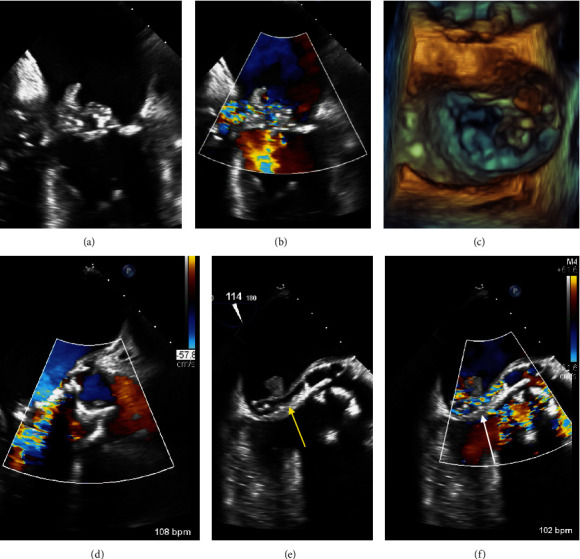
Transesophageal echocardiogram obtained approximately two weeks into hospital stay. (a–c) Bulky vegetation on the mitral valve extending along the aorto-mitral curtain with associated mitral regurgitation and possible leaflet perforation that are not well-characterized. (d) High-velocity flow in the LVOT concerning for regurgitation, as well as (e) an abnormal lucency along the aorto-mitral curtain consistent with abscess and possible fistula (yellow arrow). (f) There was high-velocity flow associated with the abscess by color Doppler (white arrow); however, in the context of tachycardia and adjacent LVOT flow, it was difficult to discern the timing of flow in the cardiac cycle.
